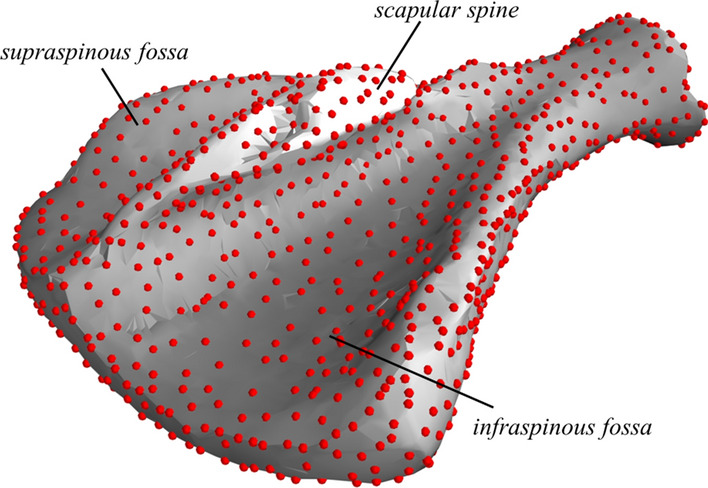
A dense surface mesh (shown in grey) of a CT-scanned purebred Norwegian Landrace scapula with semi-landmarks (shown as red dots)To reduce the computational load and to avoid problems with multiple point correspondence, when fitting semi landmarks (see (b) in “Point correspondence” below), a sparse subset of surface nodes was selected. This was done by going through all the nodes in the dense surface mesh and calculating the local volume of the sub-mesh (i.e. the mesh consisting of the convex hull of each node and the other nodes that shared faces with this node). For sub-meshes with volumes smaller than a certain threshold, the centre node was removed and a new local mesh was built. After removing nodes that were associated with the smallest local volumes, the remaining nodes constituted the scapula atlas. By doing this, the number of surface points was reduced from 7472 to 1234 semi-landmarks (red dots in Fig. 1).
